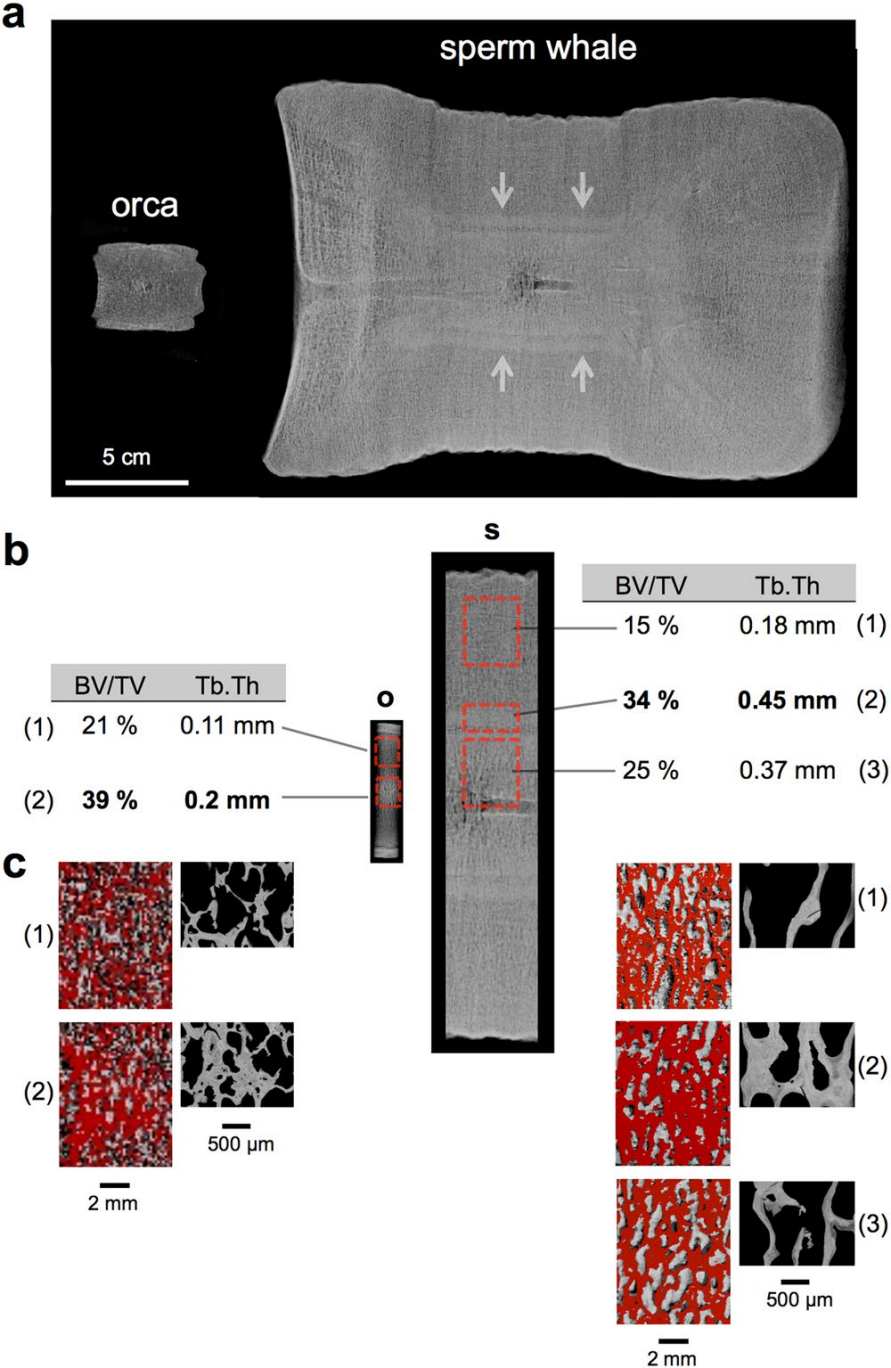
Central bone densification (bone-within-bone) in orca and sperm whale. (a) Orcas and sperm whales show a central ossification zone with contact radiography (midsagittal slice of 0.8 cm). Note the dimensions of the vertebral bodies. (b) Punch biopsies from endplate to endplate were taken. In the orca (o) and sperm whales (s), further quantification of the different regions of interest (red boxes) using HR-pQCT revealed an increased bone volume fraction (BV/TV) with thicker trabeculae (Tb.Th) in the central parts, and it corresponded to the “bone-within-bone” structure seen in radiographies. (c) Representative images of the scanned subregions in HR-pQCT indicate that the trabecular network was denser in the central parts.
